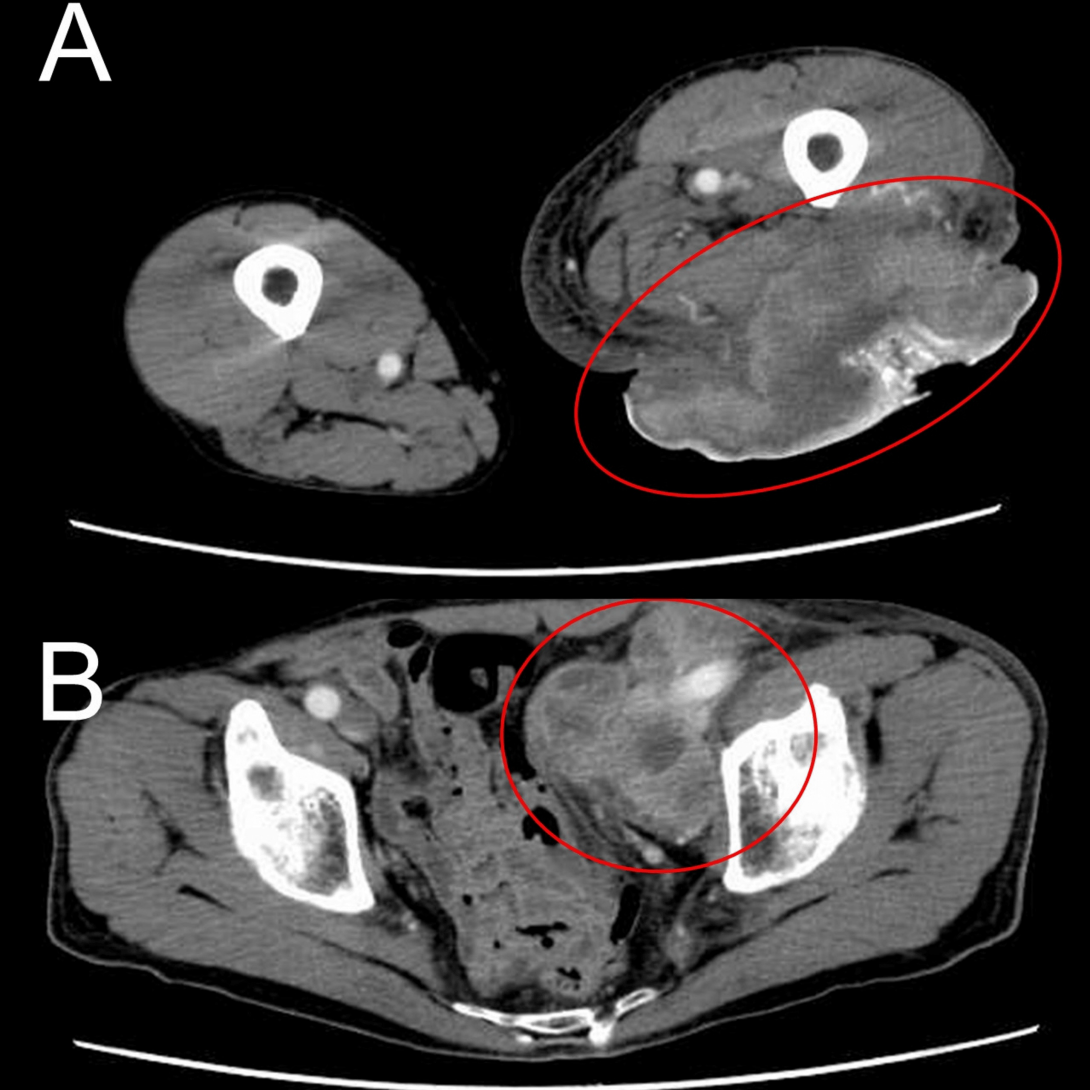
CT imaging findings (A) A contrast-enhanced CT image showing the large mass in the posterior left thigh, with areas of enhancement and necrosis. The tumor and necrotic areas are indicated. (B) A CT image showing multiple enlarged lymph nodes from the left external iliac to the para-aortic regions.
